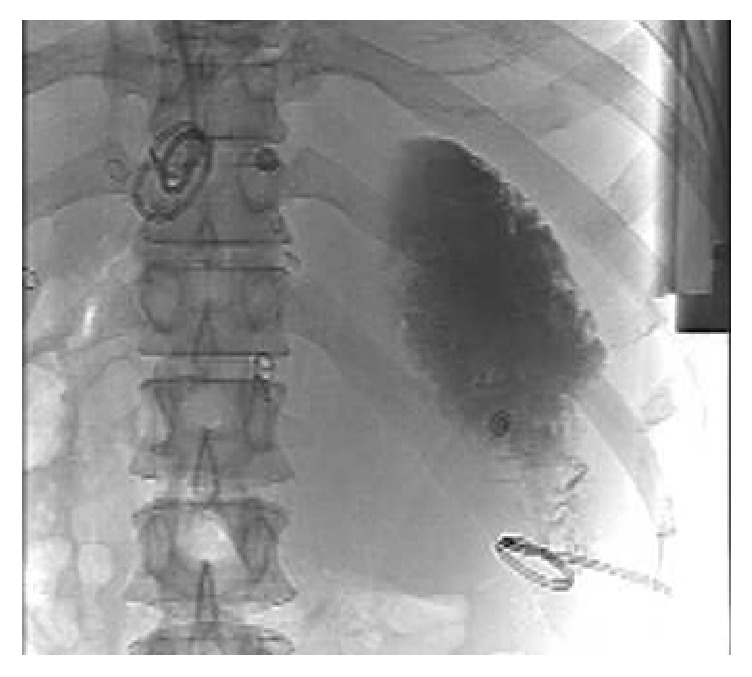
After US-guided percutaneous puncture drainage of pancreatic pseudocyst, X-ray showing drainage tube was in the right place.
